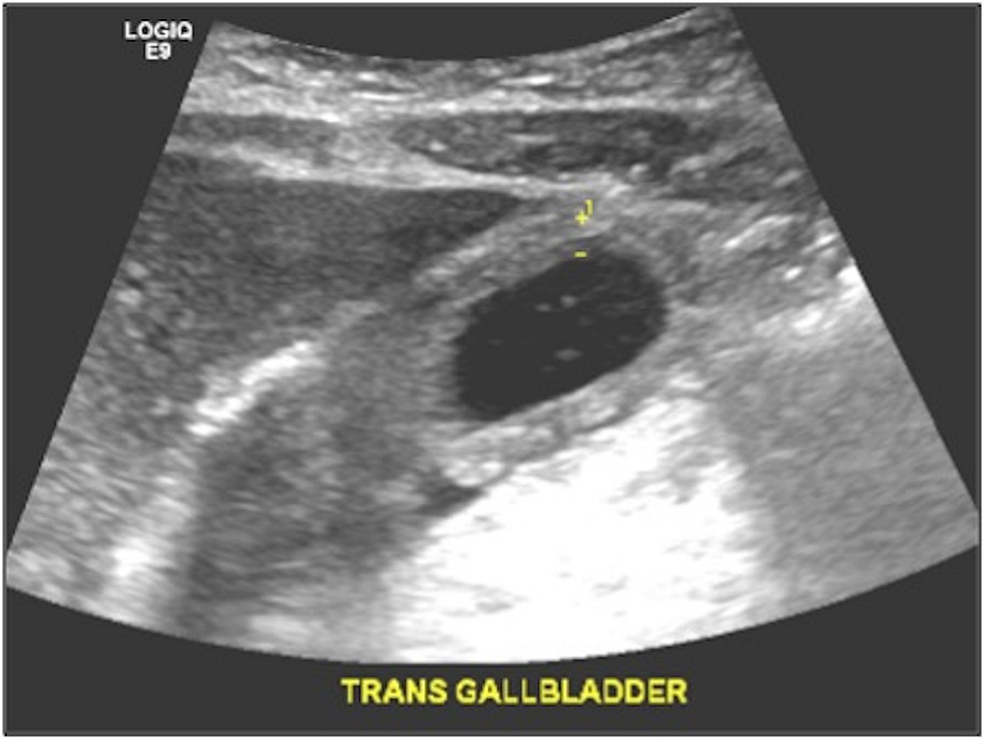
Gallbladder ultrasound showing no evidence of gallstones, but demonstrating gallbladder wall thickening, and pericholecystic fluid.
